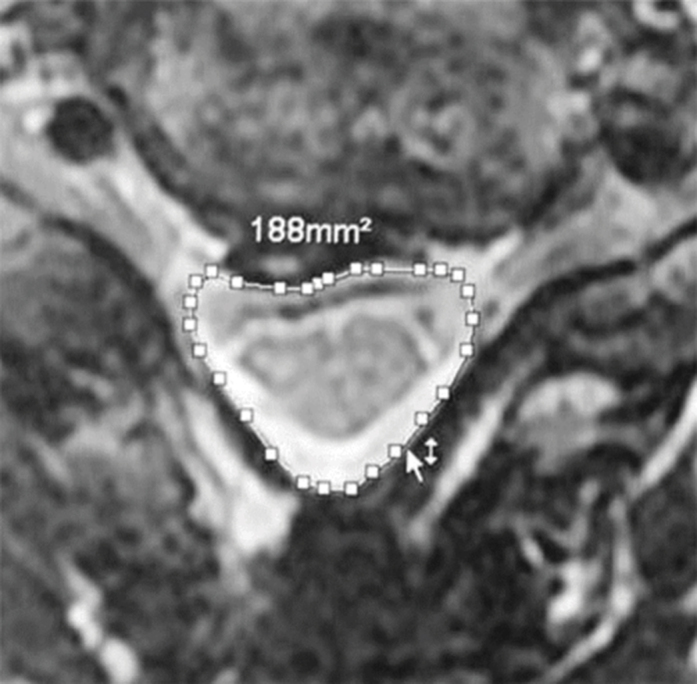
Axial T2-weighted magnetic resonance image showing the measurement of the postoperative cross-sectional areas of the dural sac.
